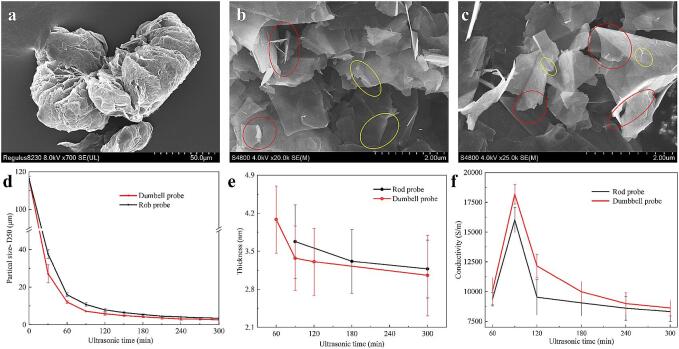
SEM images: a, expanded graphite raw material; b and c, after 300 min of ultrasound, the FLG was prepared by the rod and dumbbell probe. Red marks indicate rippled, crumpled, and curled areas; yellow marks highlight regions with crease lines and wrinkles. Evolution of FLG characteristics with increasing ultrasonic time: d, particle size(D50); e, thickness; f, the conductivity of FLG-CMC films. (For interpretation of the references to colour in this figure legend, the reader is referred to the web version of this article.)
